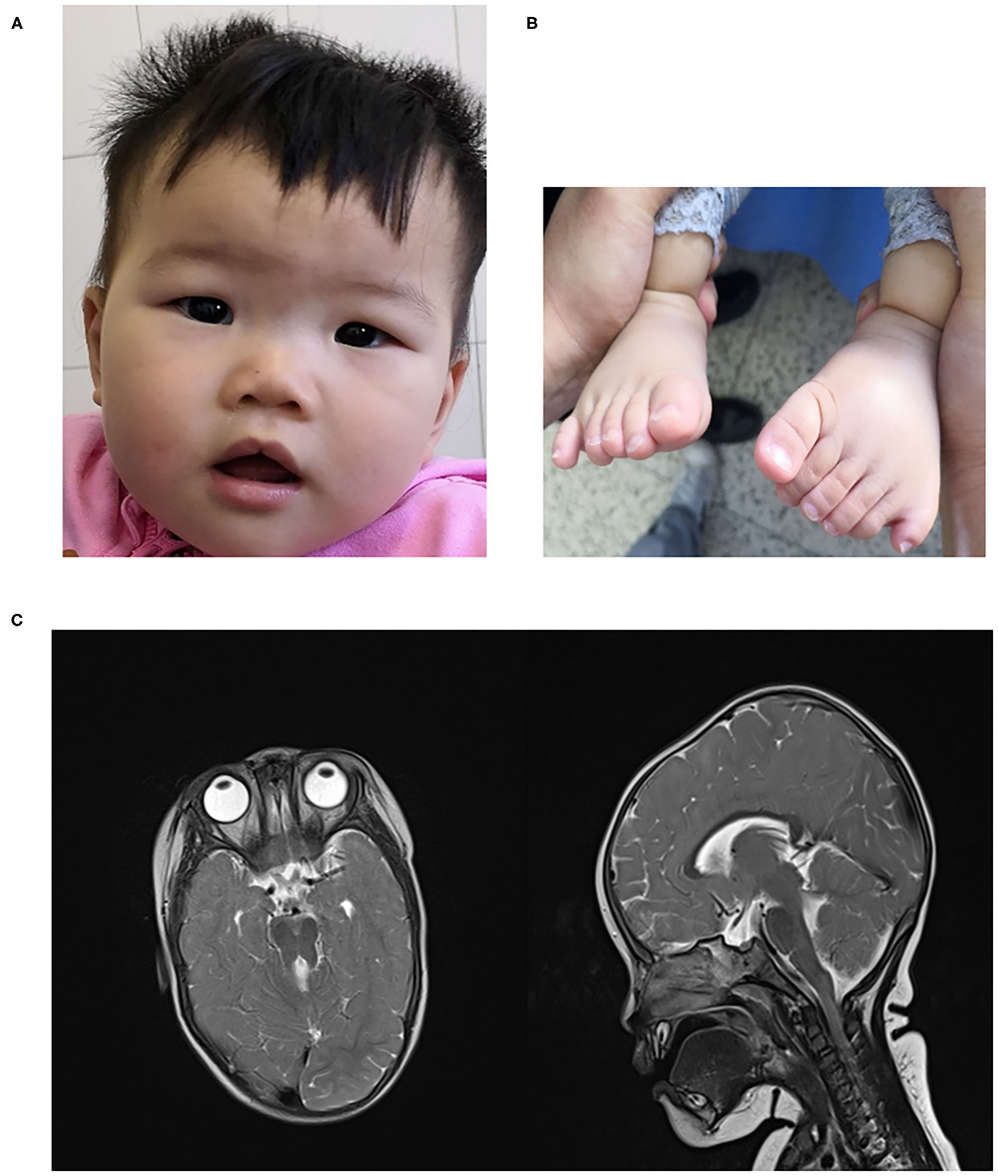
(A) craniofacial dysmorphism (B). polydactyly (C) MTS in brain MRI.
